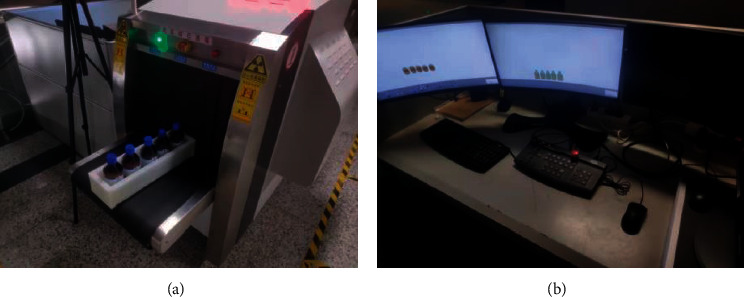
(a) The X-ray security inspection equipment. (b) The control station.
